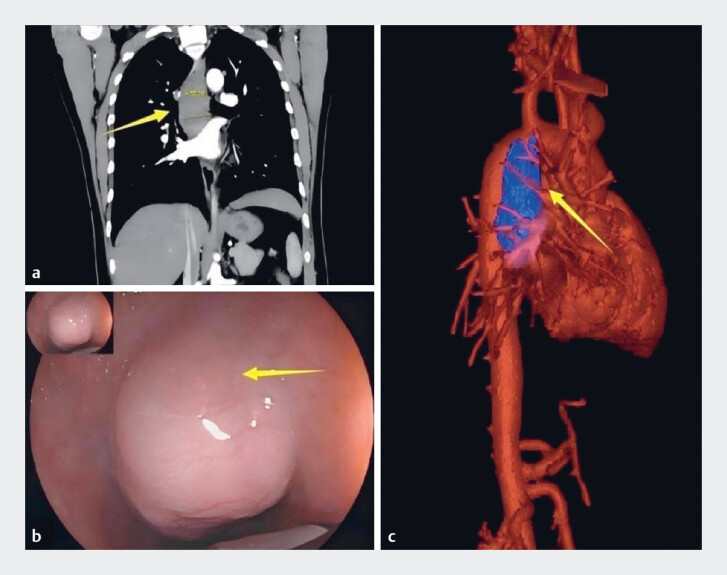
Computed tomography (CT) and endoscopy images of the lesion (arrow).
